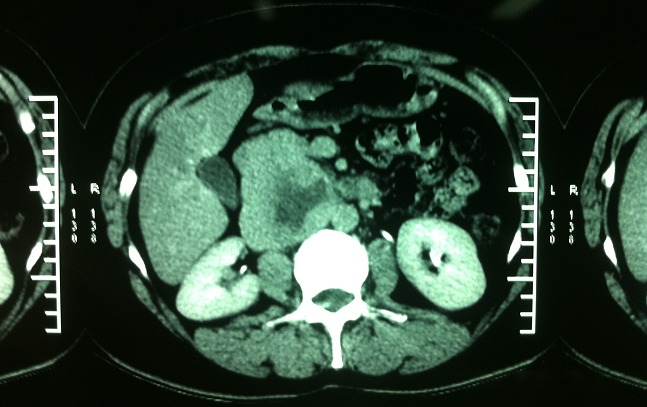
Axial CT scan image showing a round mass with a necrotic center, touching the aorta and inferior vena cava
